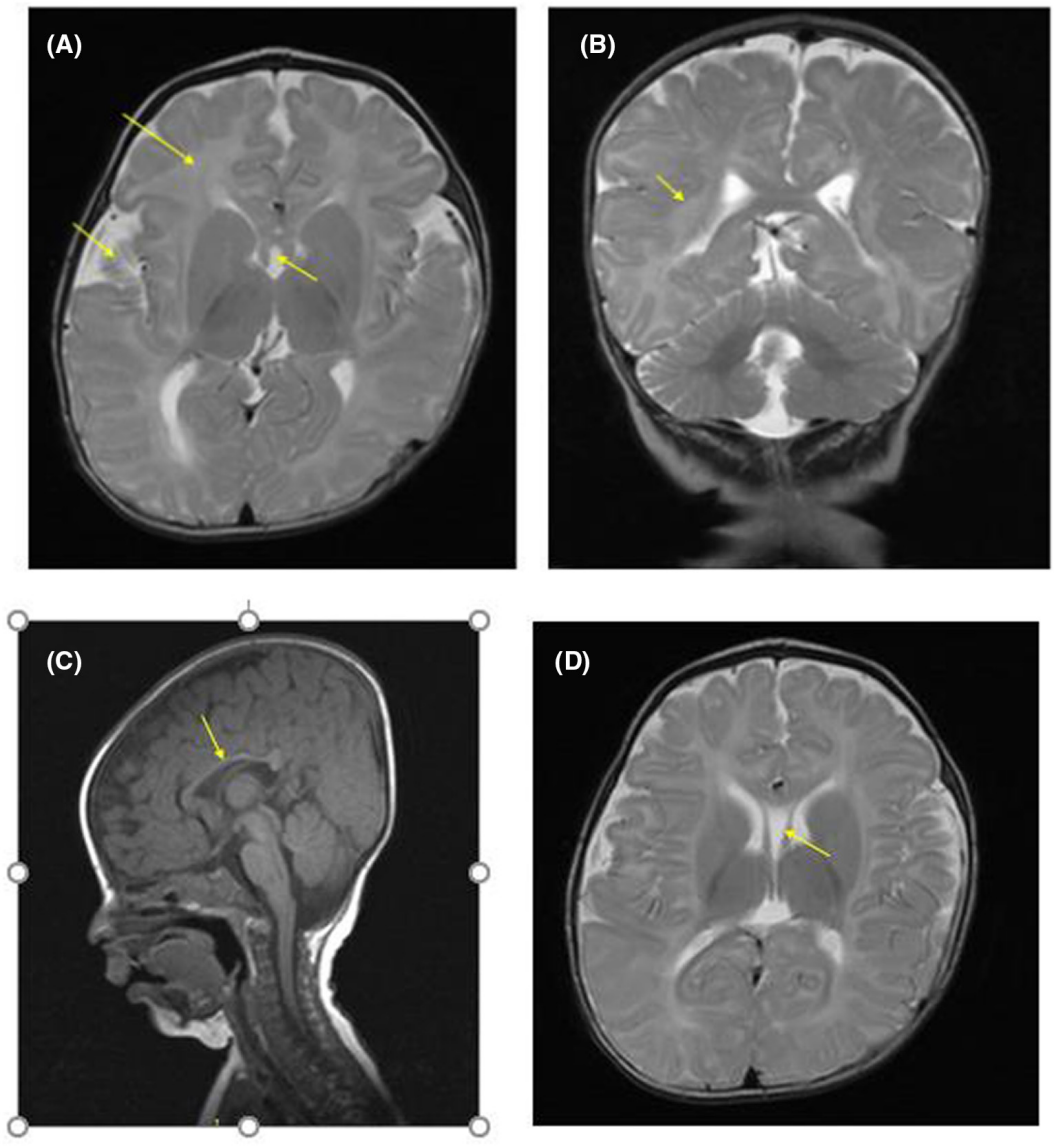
Brain MRI scan for patient 2 showing delayed myelination (A, uppermost arrow and B), enlarged sylvian cistern (A, left arrow), cavum septum pellucidum (A, right arrow and D), hypoplastic (thin) corpus callosum and trigonocephaly (C).
